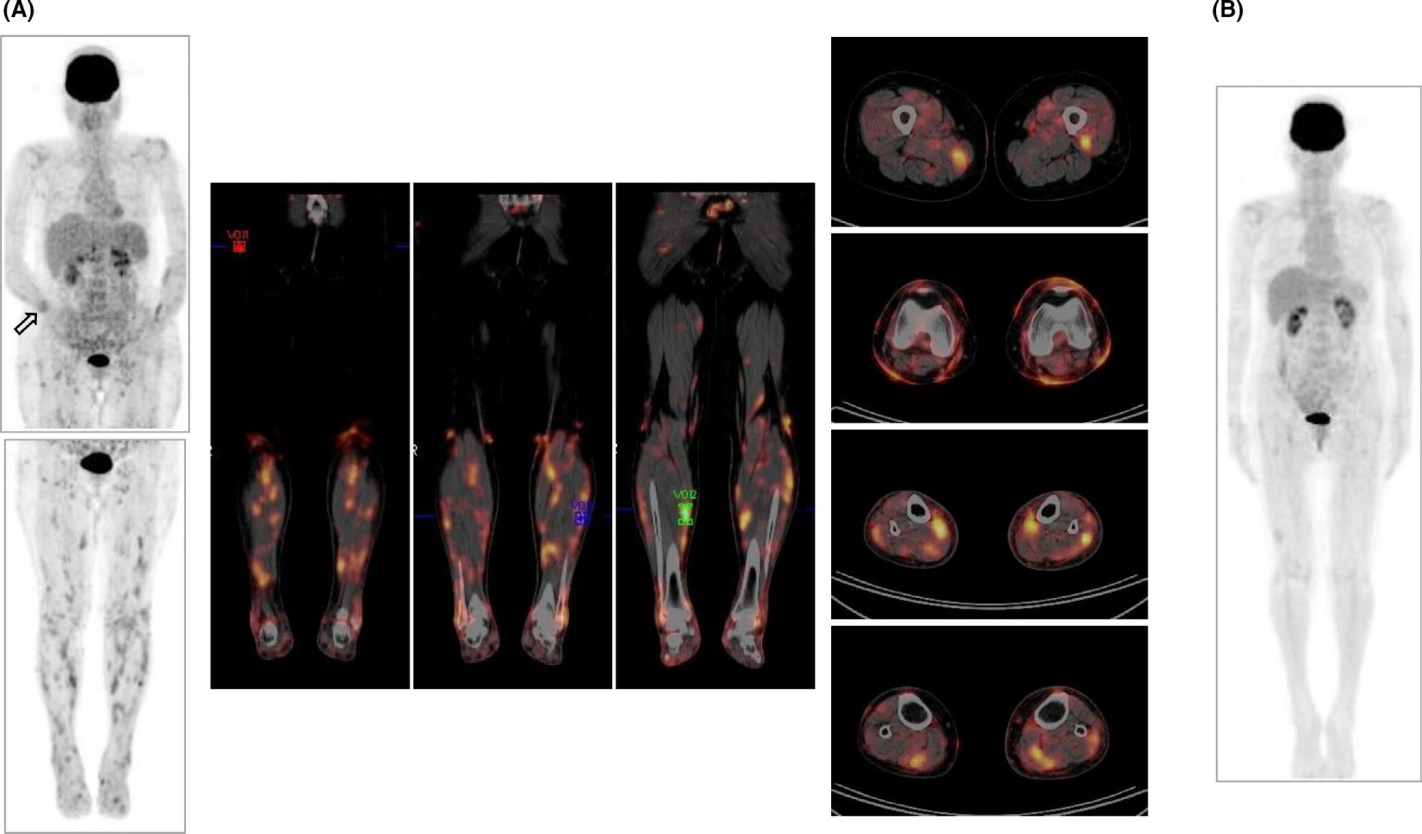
^18^F‐FDG‐PET/CT. (A) Images obtained before the initiation of chemotherapy. Anterior view of maximum intensity projection (MIP) images of the body (*left*) and representative coronal (*middle*) and axial (*right*) PET/CT fusion images of the legs are shown. The site of the radial fracture is indicated by an arrow. The maximal standardized uptake value of the muscular lesions was 5.16. (B) Anterior view of an MIP image of the body obtained on day 49 of haplo‐HSCT
